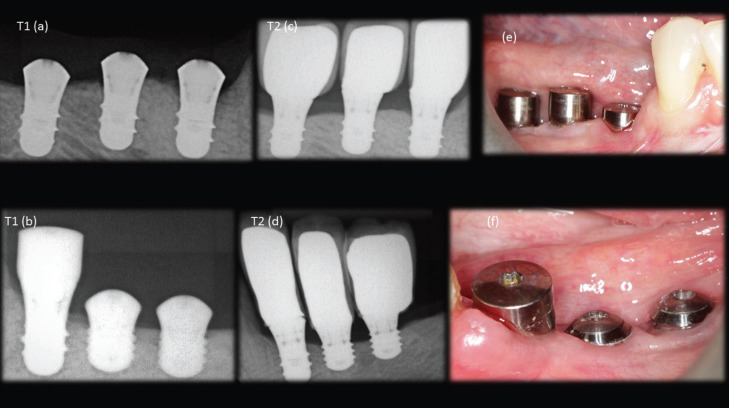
a,b) immediate x-ray of the implants installed; c,d) radiograph after one year of masticatory function; e,f) clinical photography.
